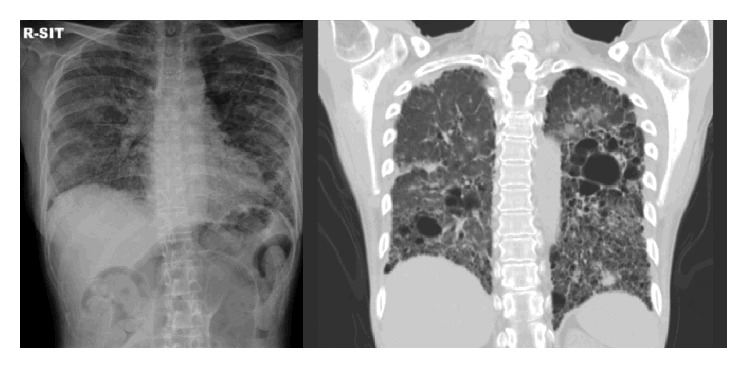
Chest radiography and CT findings at the emergent admission. Diffuse ground glass or reticular shadow with honey combing appearance in both lung fields.
